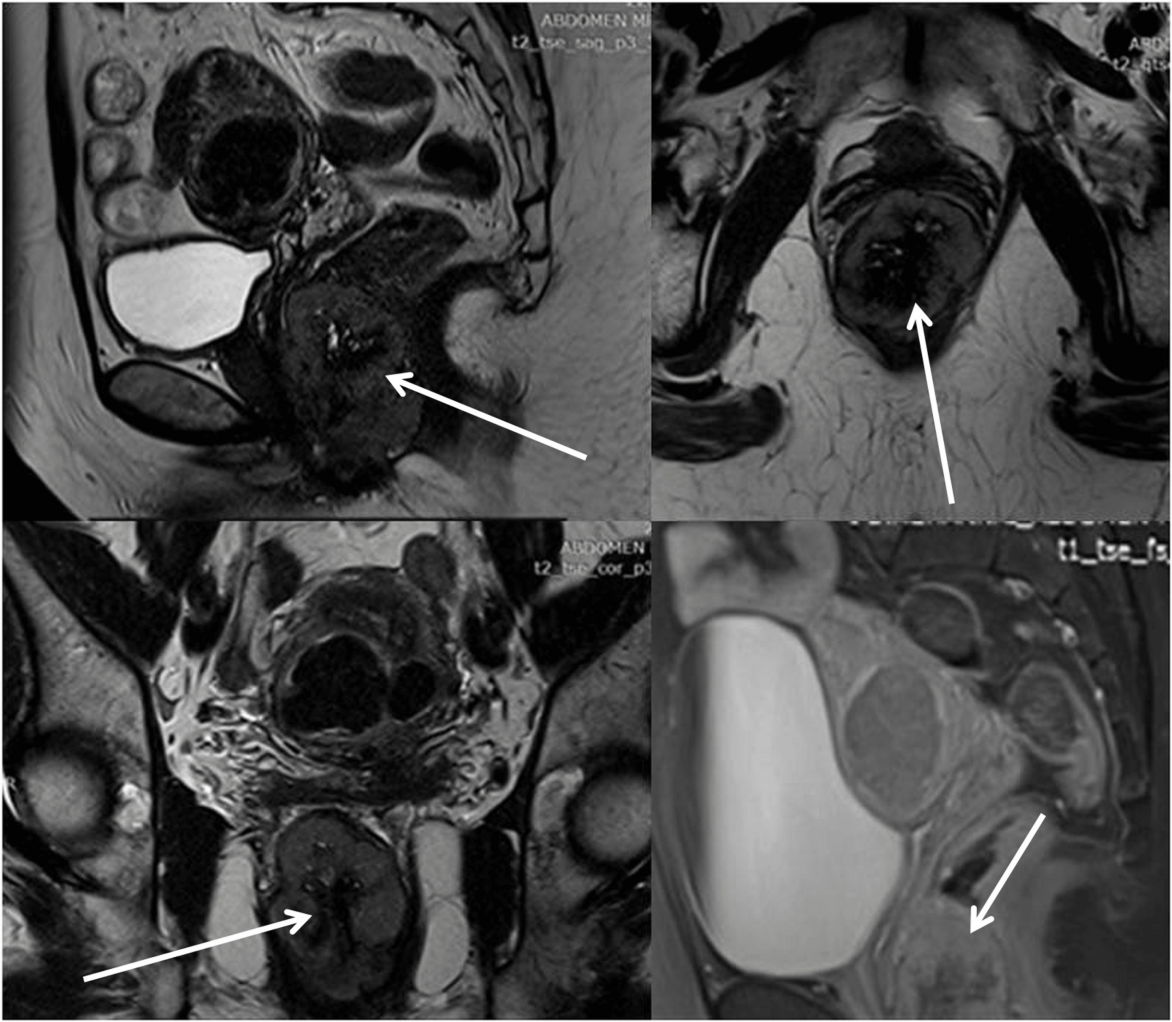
Abdominal and pelvic MRI revealed a sizeable compact lesion (arrows) between the dorsal area of the vagina, cervix, and rectum (5.9 cm craniocaudal, 4.2 cm transverse, and 4.2 cm anteroposterior diameter) It demonstrates moderate hypervascularity, especially at its intensively compact portion and it seems to compress the nearby intestines. MRI, magnetic resonance imaging
